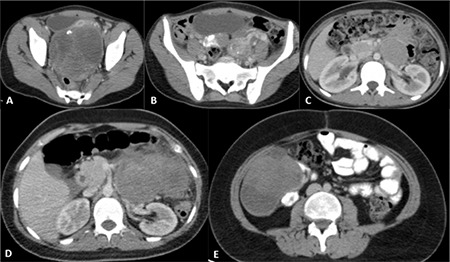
A huge mass with internal cystic components and calcifications, within the cul de sac. Note that the bladder and uterus are displaced anteriorly and the rectum is displaced posteriorly by the lesion (A). Another lesion with calcifications. A small cyst is also visible at the upper level (B), retroperitoneal lymphadenopathy with mild compression on the left renal vein (C). Axial computed tomography image at the renal sinus level shows a huge mass with irregular borders and heterogeneous enhancement within the left retroperitoneal localization, anterior to the left kidney (D). A hypo dense mass with mild irregular contours in the right abdomen at the posterolateral aspect of the caecum and ascending colon (E)
